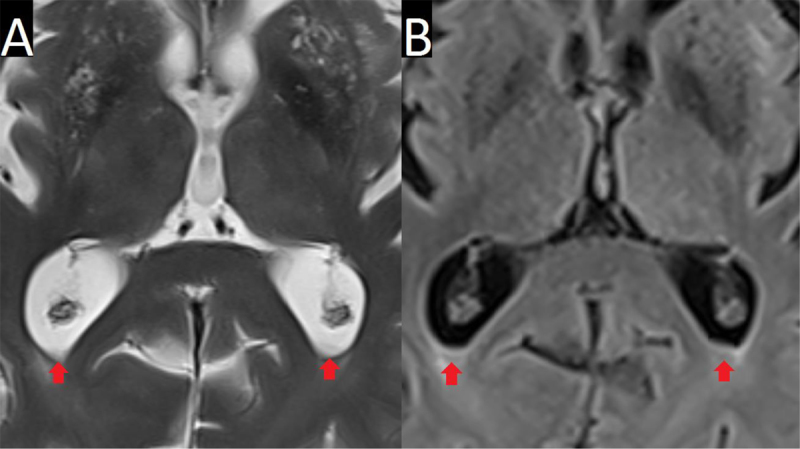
MRI showed a bright spot in the subarachnoid space of the right parietal region on DWI (Figure 3B) appearing slightly hypointense on FLAIR (Figure 3A).
